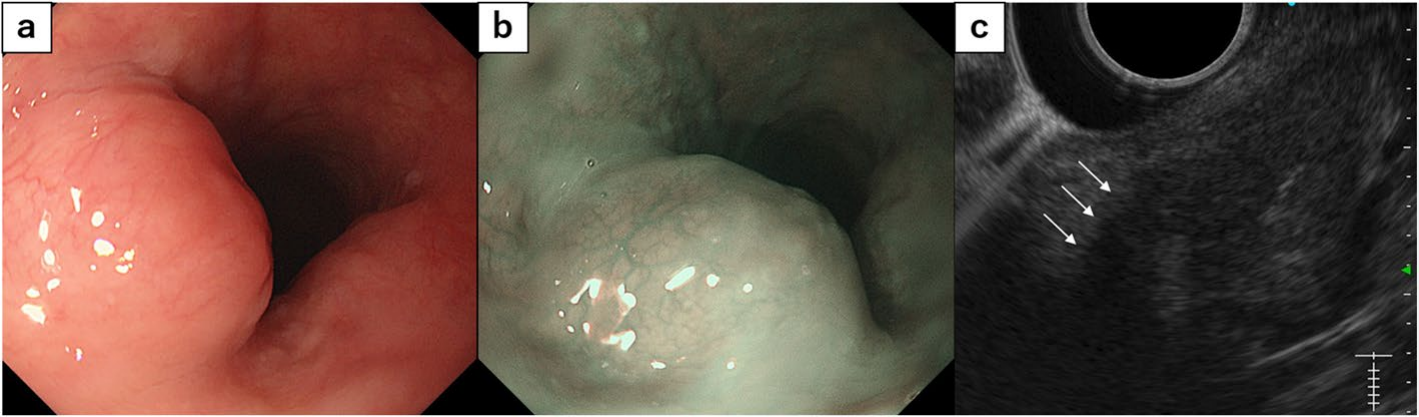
Upper gastrointestinal endoscopic findings. a An elevated lesion covered by normal mucosa was observed in the left wall of the cervicothoracic esophagus 16 cm from the incisor. Approximately one-quarter of its circumference was visible. b Observation with NBI (narrow-band imaging). c Ultrasonographic findings. A hypoechoic area with a maximum diameter of 16 mm was observed at the same site. The lesion was located in the intrinsic muscularis propria. Its interior was heterogeneous, its margins were irregular, and the boundary between the muscular layer and perimural tissue was unclear (white arrows)
